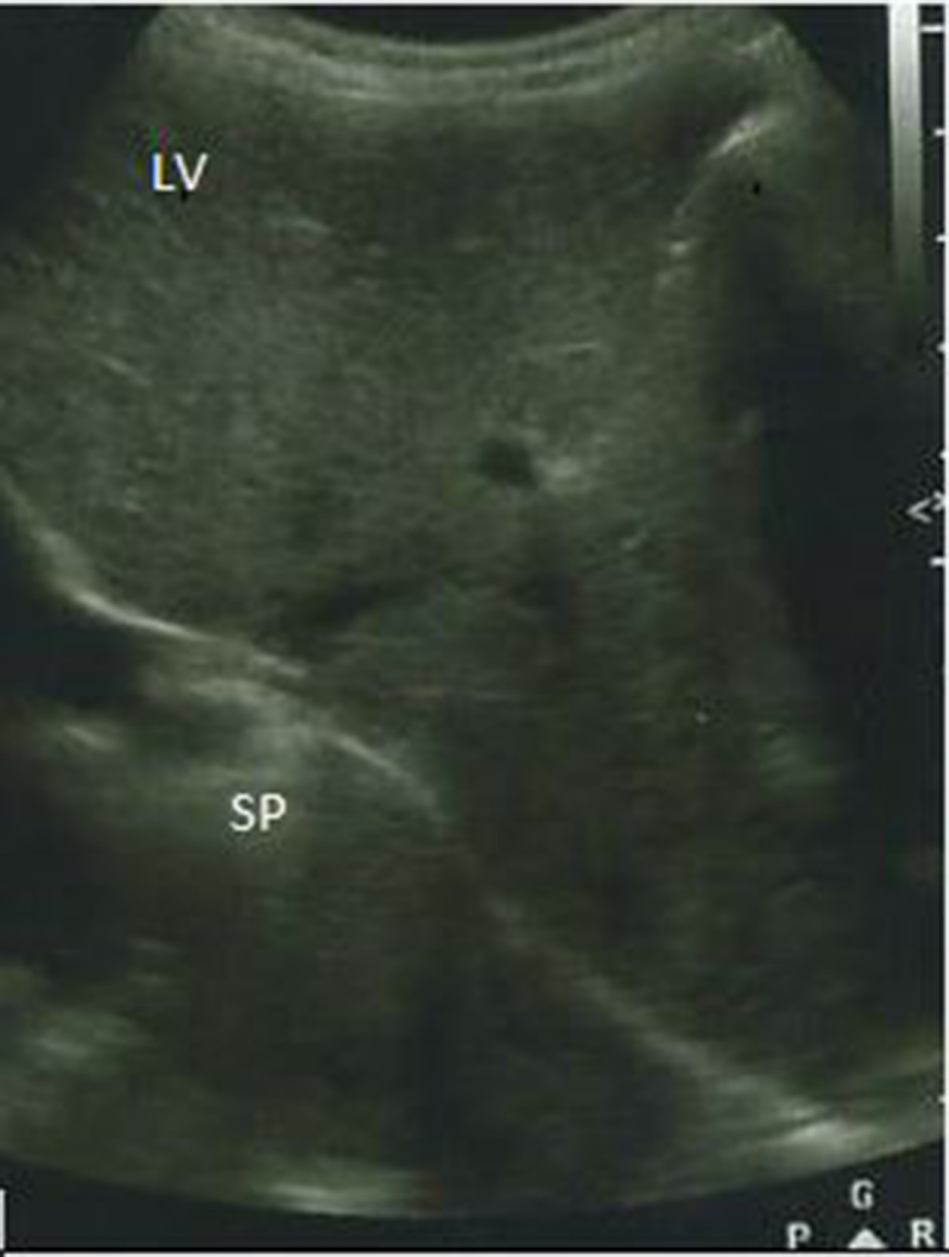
Ultrasound scan showing the SP adjacent to the LV. SP, spleen; LV, liver.
